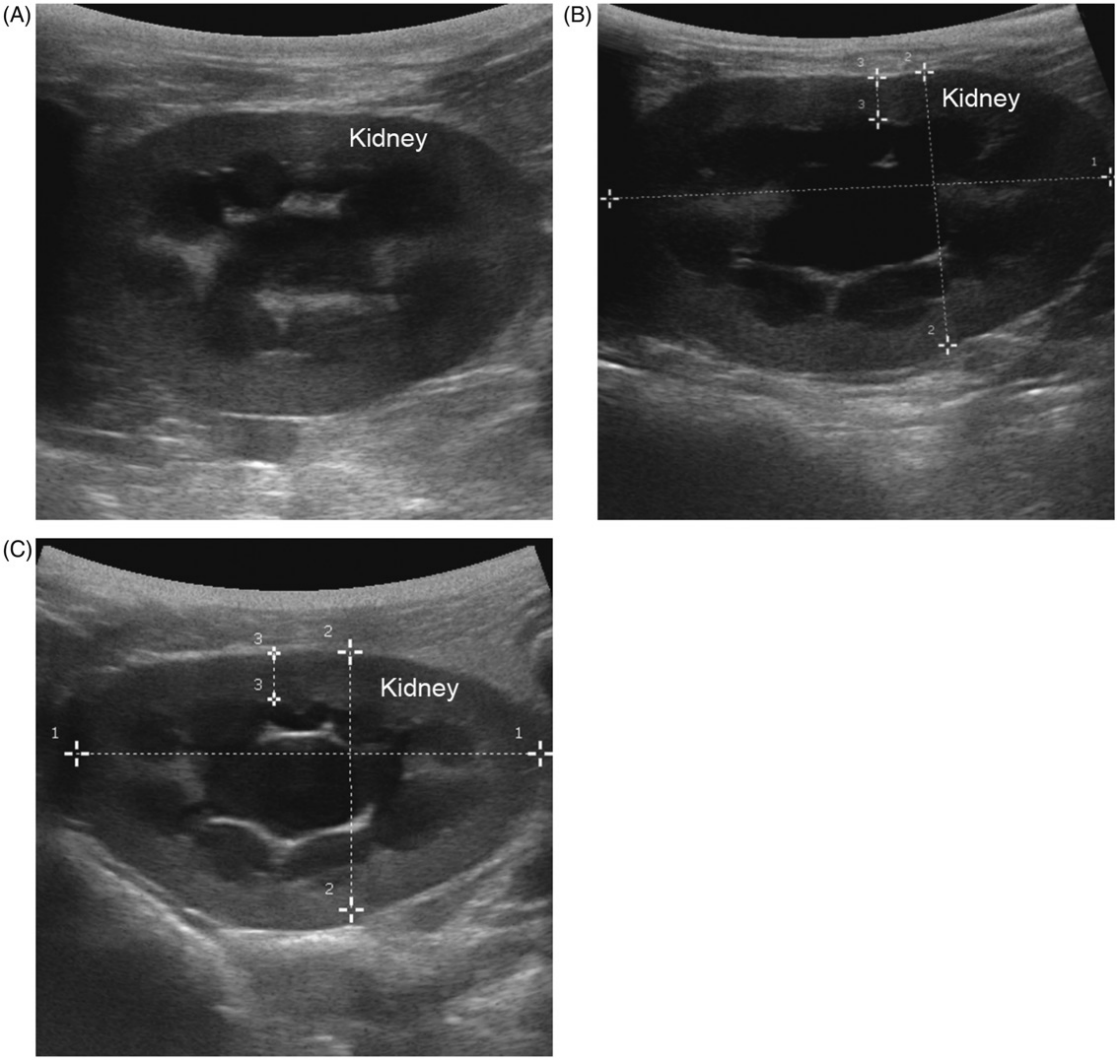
Postoperative ultrasonography of the kidney. (A) Ultrasonography of kidney in shame control group on the 10th day postoperatively. (B) Ultrasonography of kidney in PUUO group on the 10th day. The RCT decreased, while V increased into hydronephrosis. (C) Ultrasonography of kidney in RUUO group on the 20th day after relief.
